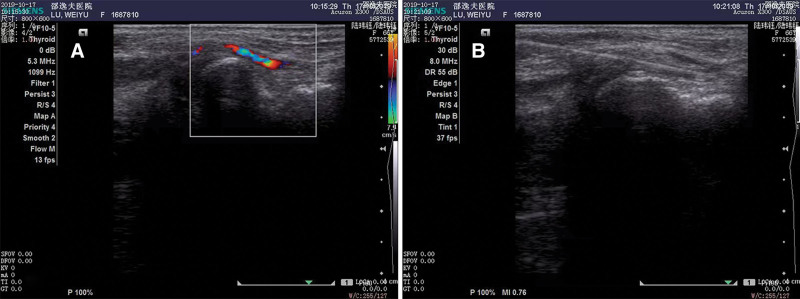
Ultrasound was used to help distal radial artery access. (A) The long-axis ultrasound images of the distal radial artery showed a color flow signal. (B) Under the guidance of ultrasound, the left DRA was successfully punctured with a 20-gage needle. DRA = distal radial artery.
